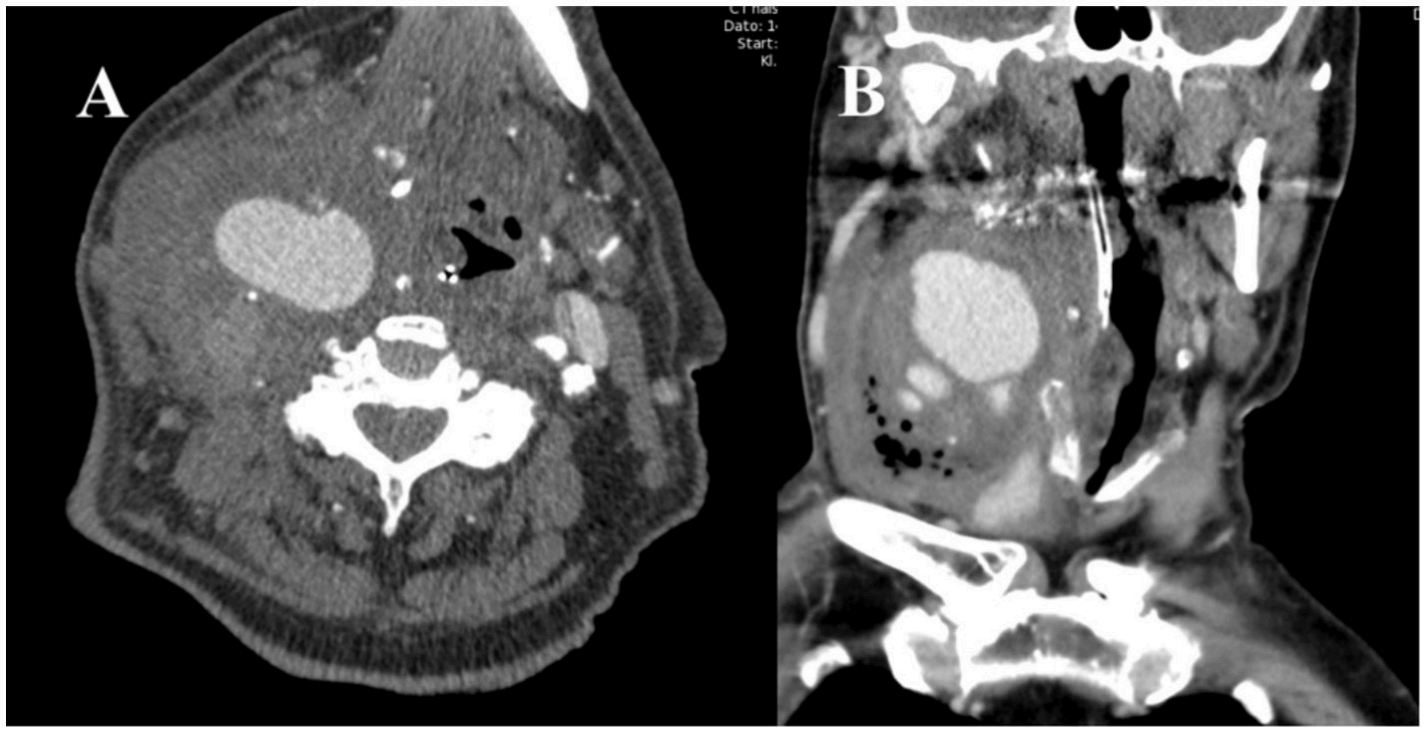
CT-angiography showing contrast in a now dilated, growing IA. (A) Transverse plane (B) Coronal plane.
